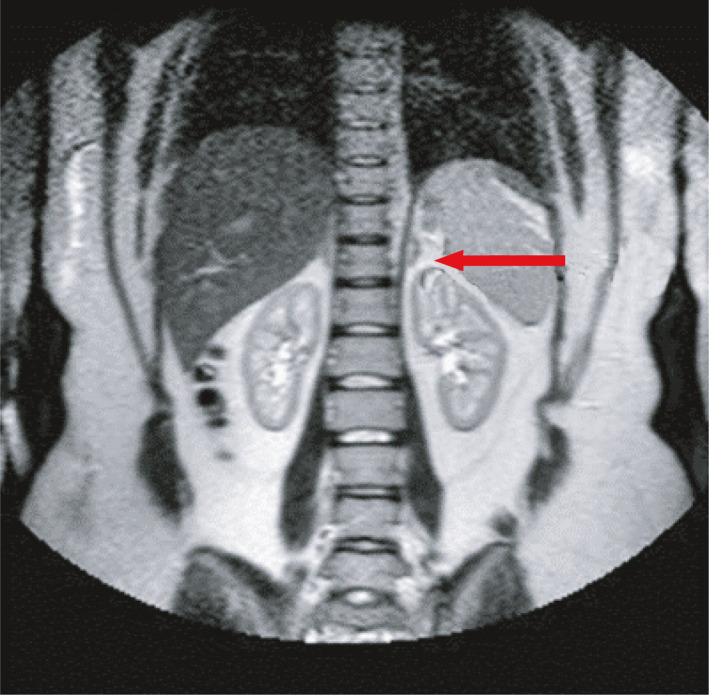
MRI abdomen in the axial view showing a normal left adrenal gland
